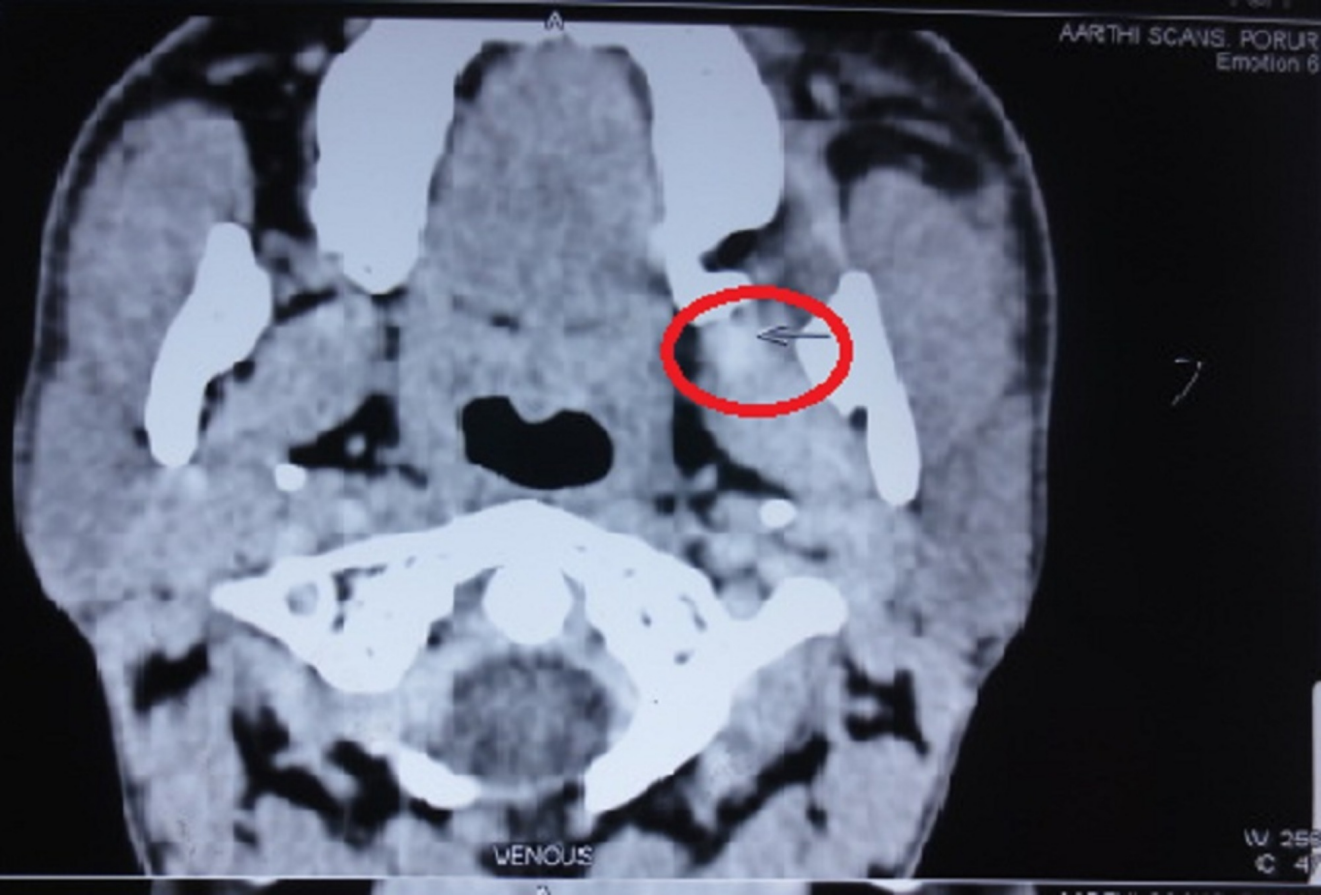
Computed tomography (CT) scan Red circle showing clinically enhancing lesion in the left retromandibular region with adjacent mandibular erosion.
